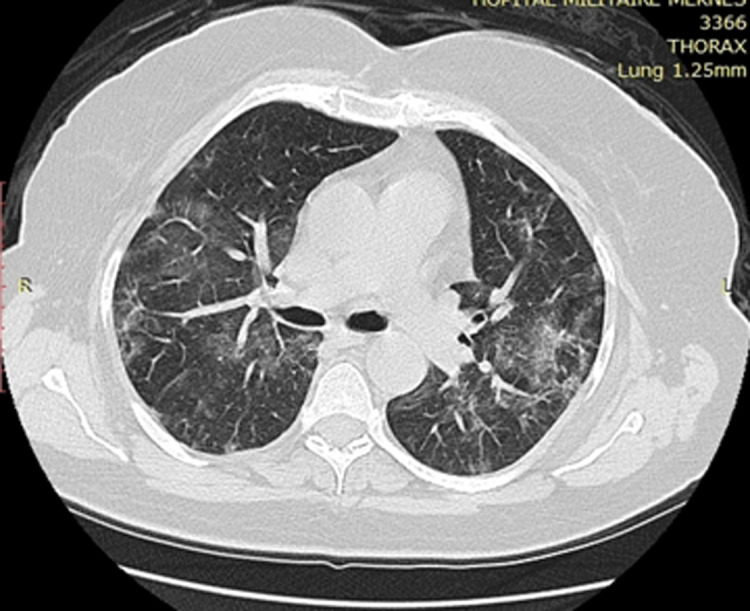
control chest computed tomography
